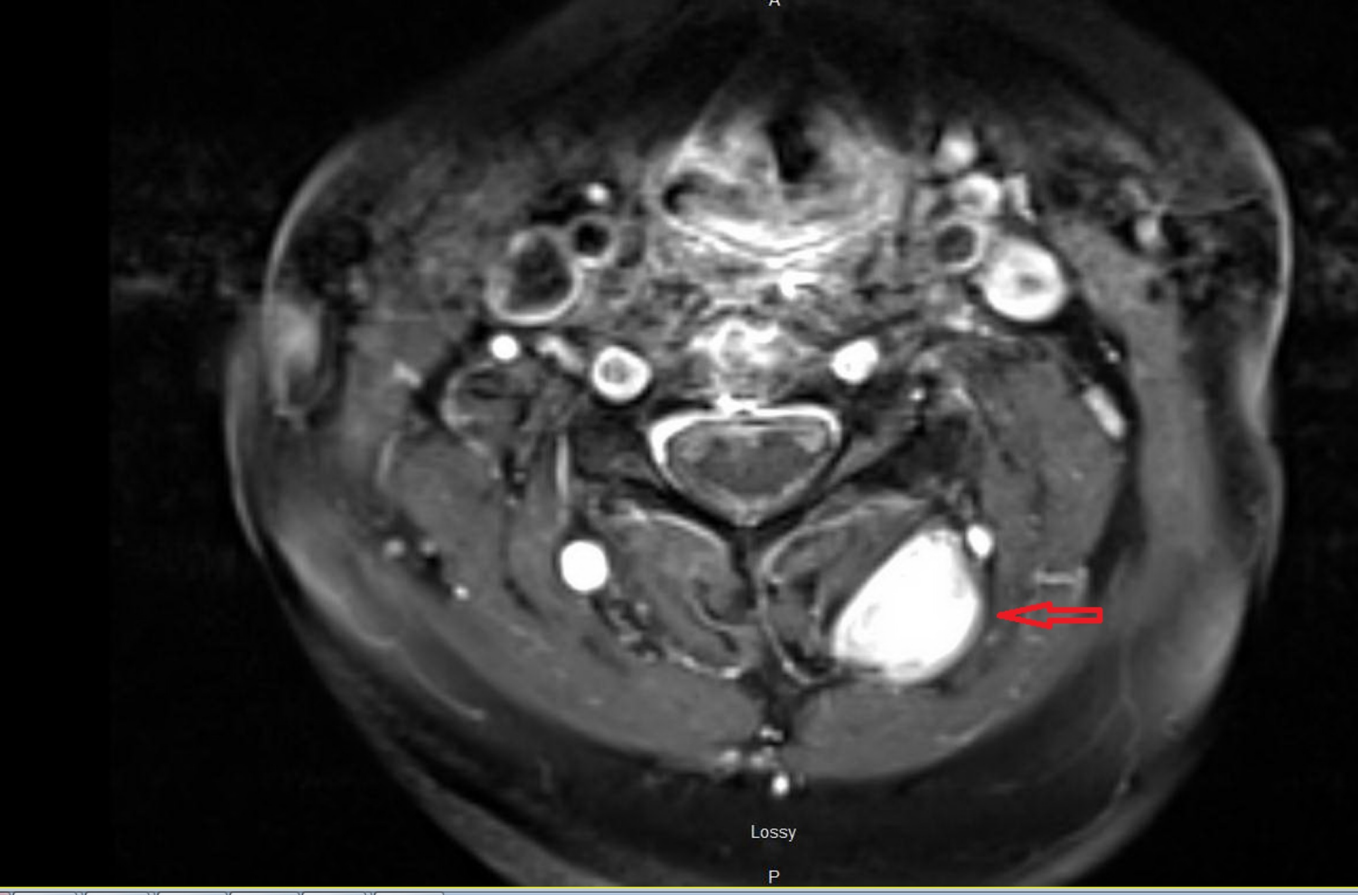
Axial MRI postcontrast. Postcontrast MRI showing enhancement of the mass in the axial plane.
